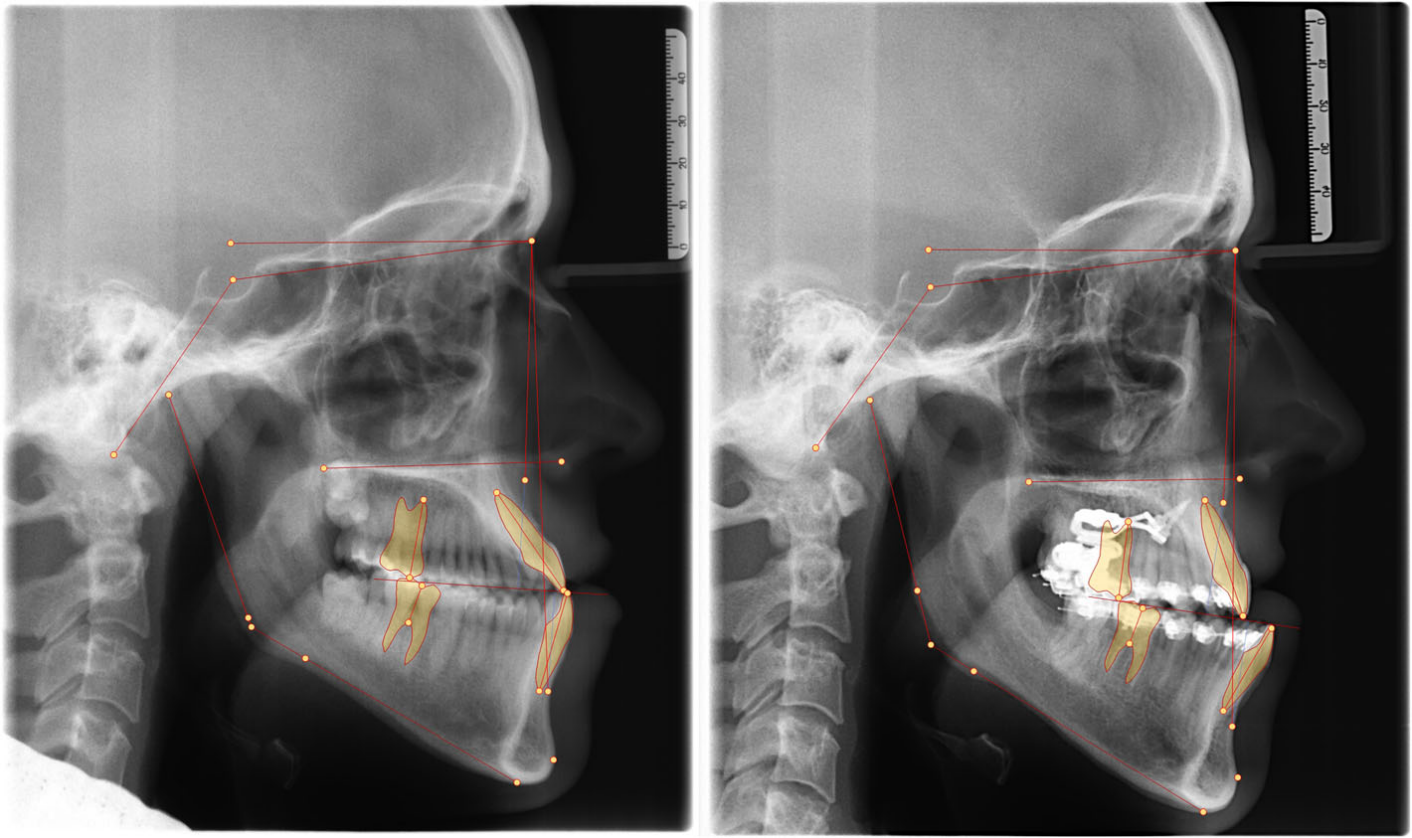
Radiograph comparison before orthognathic surgery: lateral cephalogram pre- (left) and post-distalization (right)
